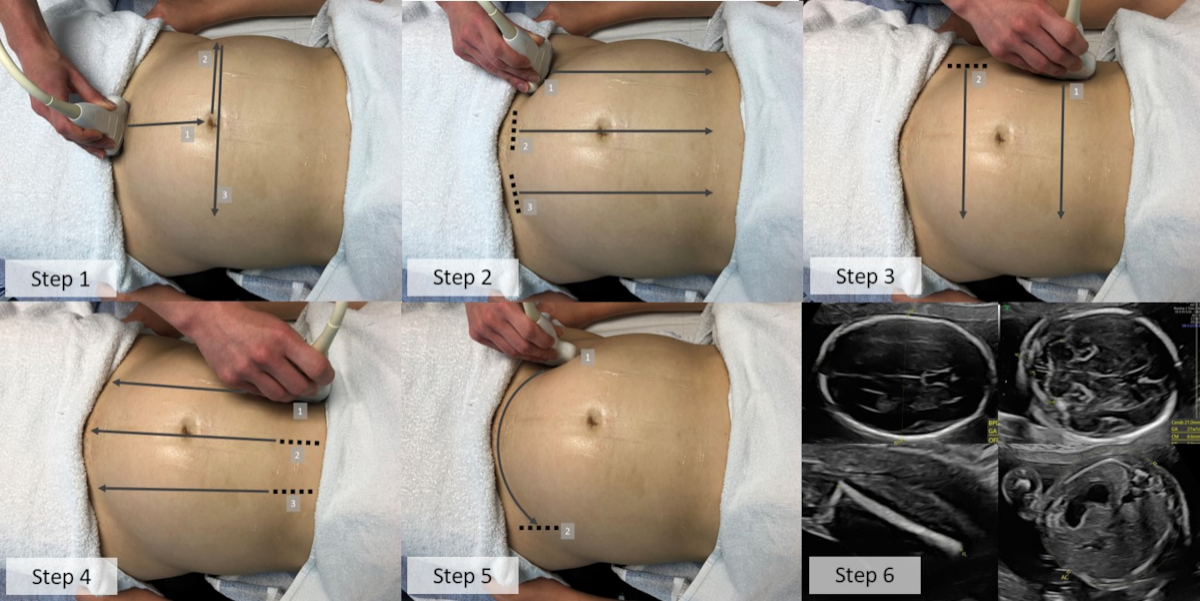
The Computer-Assisted Low-Cost Point-of-Care UltraSound protocol, developed on the basis of the studies described (see text).
